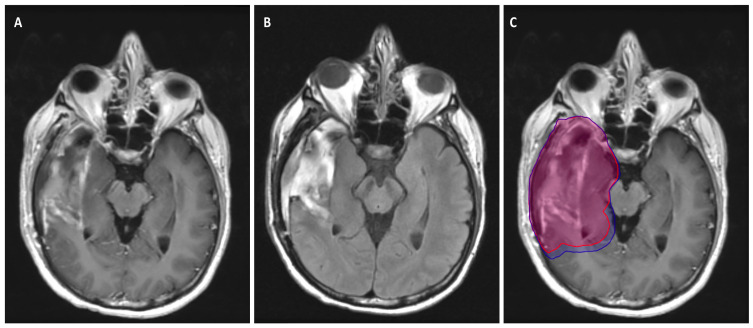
A patient who was diagnosed with a right temporal lobe GBM. He underwent gross total resection and was subsequently treated with 60 Gy in 30 fractions with concurrent TMZ, followed by adjuvant TMZ. The RTOG approach was used to generate the target volumes. (A) T1 post-contrast axial MRI taken 48 h post gross total resection with a large postoperative cavity in the right temporal lobe; (B) T2 FLAIR axial MRI at the same level as (A) demonstrating significant surrounding edema; (C) Planning scan with PTV1 and PTV2 overlaid on (A). The area encompassed by the blue volume is receiving 46 Gy in 23 fractions, while the area encompassed by the red volume is receiving an additional 14 Gy in 7 fractions to a total dose of 60 Gy in 30 fractions.
